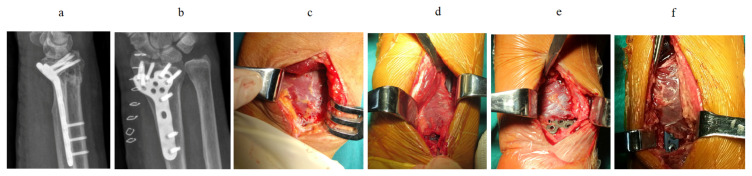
Patients whose pronator quadratus was preserved. a) one case of type C fracture was demonstrated by lateral radiography one week after operation. b) Anterior and posterior radiographs of 1 case of type B fracture one week after operation. c) Display of findings in 1 case of type C fracture after plate implantation. d) Display of findings in 1 case of type B fracture after plate implantation. e) Muscle condition of 1 case of type C fracture when the steel plate was removed in the second operation. f) Muscle condition of 1 case of type B fracture when the steel plate was removed in the second operation.
